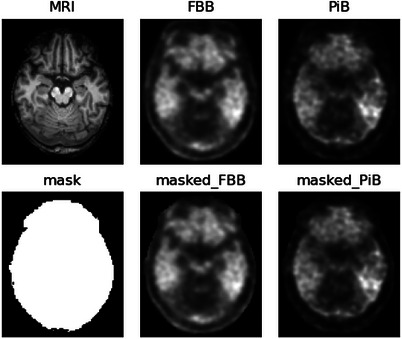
A representative subject showing the network inputs and the MRI‐based mask. MRI, medical resonance imaging.
